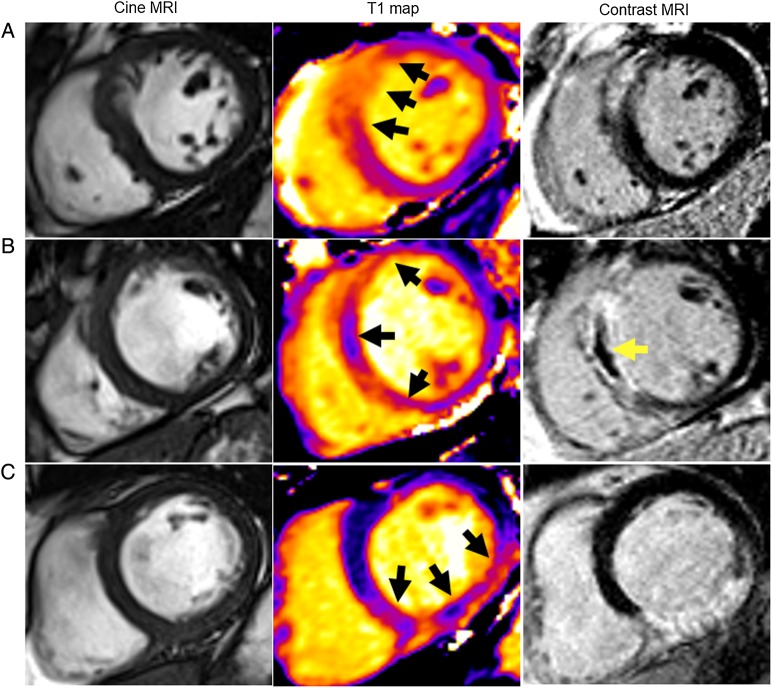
Three patients with acute ST-elevation myocardial infarction treated by primary PCI and with the same anti-thrombotic therapies, including aspirin, clopidogrel, heparin, and intravenous tirofiban. Each patient had normal thrombolysis in myocardial infarction Grade 3 flow at the end of PCI. Cardiac magnetic resonance imaging was performed for each patient 2 days later. (*A*) Patient with no T1 hypo-intense infarct core and no microvascular obstruction*.* Native T1 within the injury zone (*middle*) measured 1211 ms. Acute infarct size revealed by late gadolinium enhancement (*right*) was 22.2%. The left ventricular ejection fraction and left ventricular end-diastolic volume were 55.2% and 143.1 mL, respectively. Analysis of the repeat magnetic resonance imaging scan after 6 months follow-up indicated that the final infarct size was 15.6% of left ventricular mass and the left ventricular end-diastolic volume had reduced to 103.0 mL. This patient had an uncomplicated clinical course. (*B*) Patient with both T1 hypo-intense infarct core and microvascular obstruction*.* T1 mapping (*middle*) revealed a hypo-intense region within the infarct core, corresponding to the area of microvascular obstruction on contrast-enhanced magnetic resonance imaging (*right*). Native T1 within the infarct core measured 1036 ms, which was substantially lower than the T1 value measured at the periphery of the infarct zone (1193 ms). Acute infarct size revealed by late gadolinium enhancement (*right*) was 33.0%. Microvascular obstruction depicted as the central dark zone within the infarct territory was 3.6% of left ventricular mass. The left ventricular ejection fraction and end-diastolic volume were 45.8% and 199.3 mL, respectively. The final infarct size at 6 months was 22.6% of left ventricular mass and the left ventricular end-diastolic volume had increased to 221.8 mL. This patient was re-hospitalized for new onset heart failure during follow-up.
